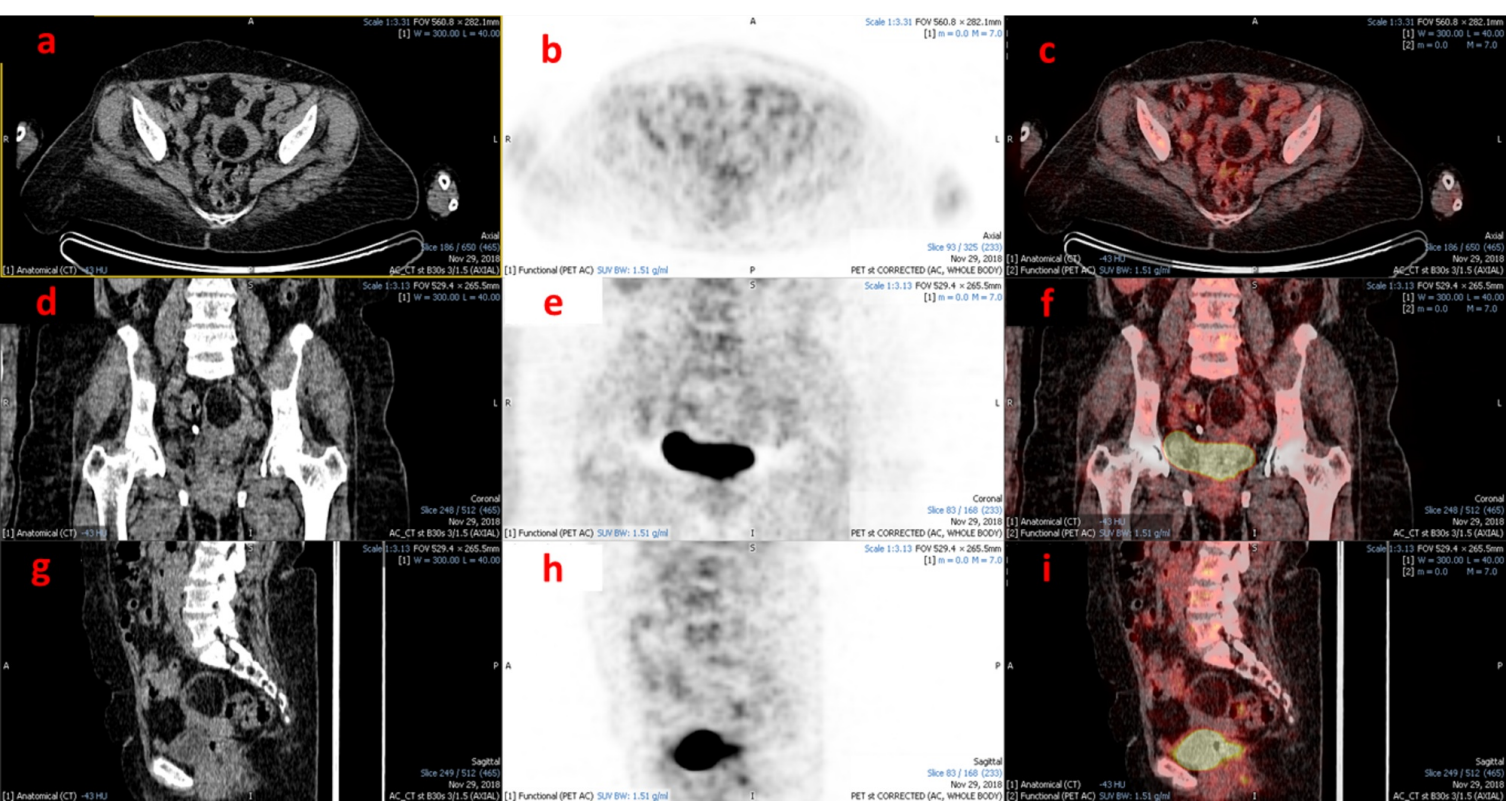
18FDG PET/CT images (a-c: axial; d-f: coronal; g-i: sagittal) showing a well-defined hypodense area (Hounsfield unit (HU): -89.9) without metabolic activity in the uterine fundus .
